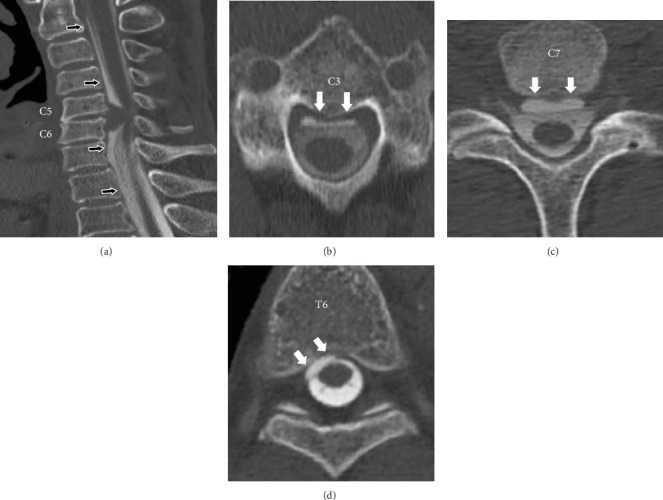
Case 1. Preoperative CT myelography. (a) Sagittal view: fluid collection on the ventral side of the dura (black arrows) adjacent to the cervical disk herniation at C5-C6. (b–d) Axial views: ventral longitudinal intraspinal fluid collection (VLISFC) between C2 and T6 (white arrows).
